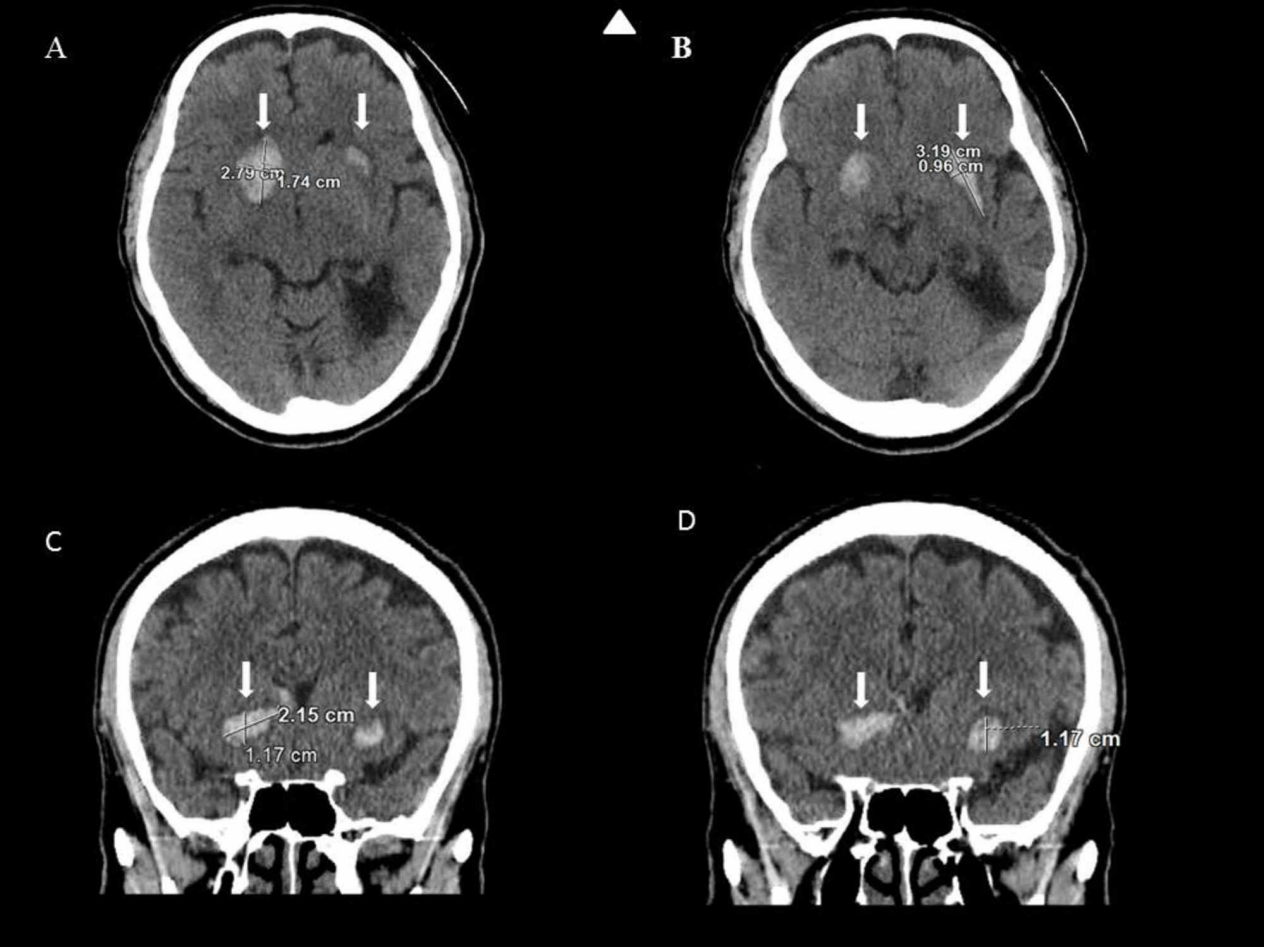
Brain CT images Brain CT shows parenchymal hemorrhage of the right basal ganglia, measuring up to 2.8 cm, with extension into the frontal horn of the right lateral ventricle. The same brain CT shows intraparenchymal hemorrhage of the left lentiform nucleus as well as chronic encephalomalacia of the left temporal lobe. Arrowhead indicates top. A small arrow indicates areas of interest, which are hyperattenuation on non-contrast CT study consistent with ICH. A: CT WO transverse; hyperattenuation measures 2.79 cm x 1.74 cm B: CT WO transverse; hyperattenuation measures 3.19 cm x 0.96 cm C: CT WO coronal; hyperattenuation measures 2.15 cm x 1.17 cm D: CT WO coronal; hyperattenuation measures 1.17cm ICH - intracerebral hemorrhage; WO - without contrast
